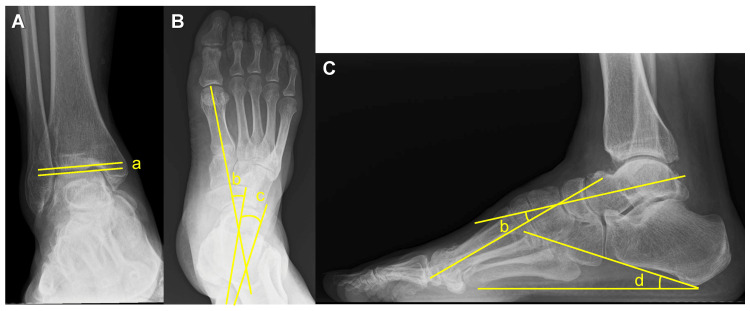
Preoperative plain radiographs on weight-bearing. (A) Anteroposterior view of the ankle; (B) anteroposterior view of the foot; (C) lateral view of the foot. (a) Talar tilt angle; (b) talo-first metatarsal angle; (c) talo-calcaneal angle; (d) calcaneal pitch angle.
